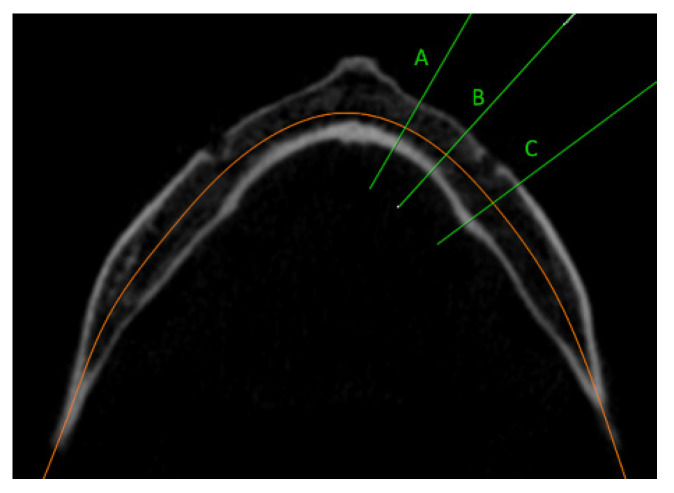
CBCT axial view of selected regions of the mandible: (A) lateral incisor region (9 mm from the midline of the mandible); (B) first premolar region (6 mm anteriorly from the middle of the mental foramen); (C) first molar region (6 mm distal from the middle of the mental foramen).
